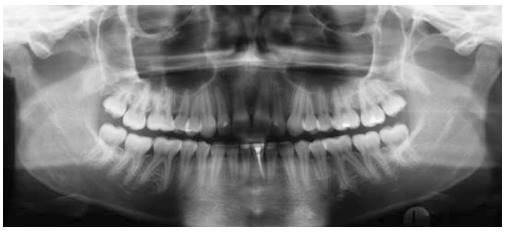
Post-treatment (T_2_) panoramic radiograph.
